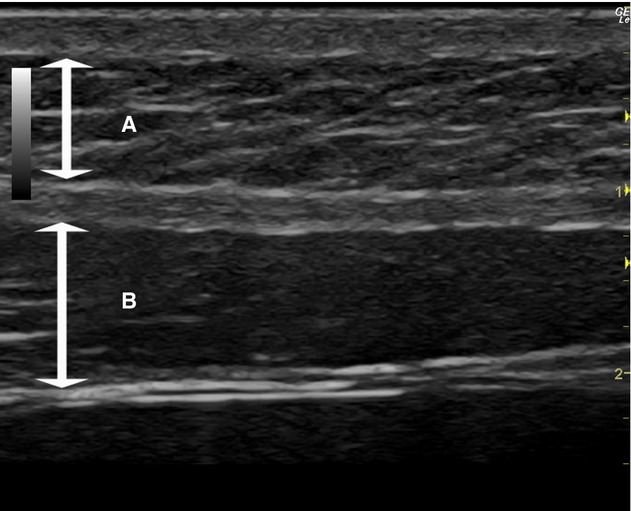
Ultrasonogram of the upper abdominal wall on the xiphoumbilical line. (A) Subcutaneous fat thickness, (B) preperitoneal fat thickness.
